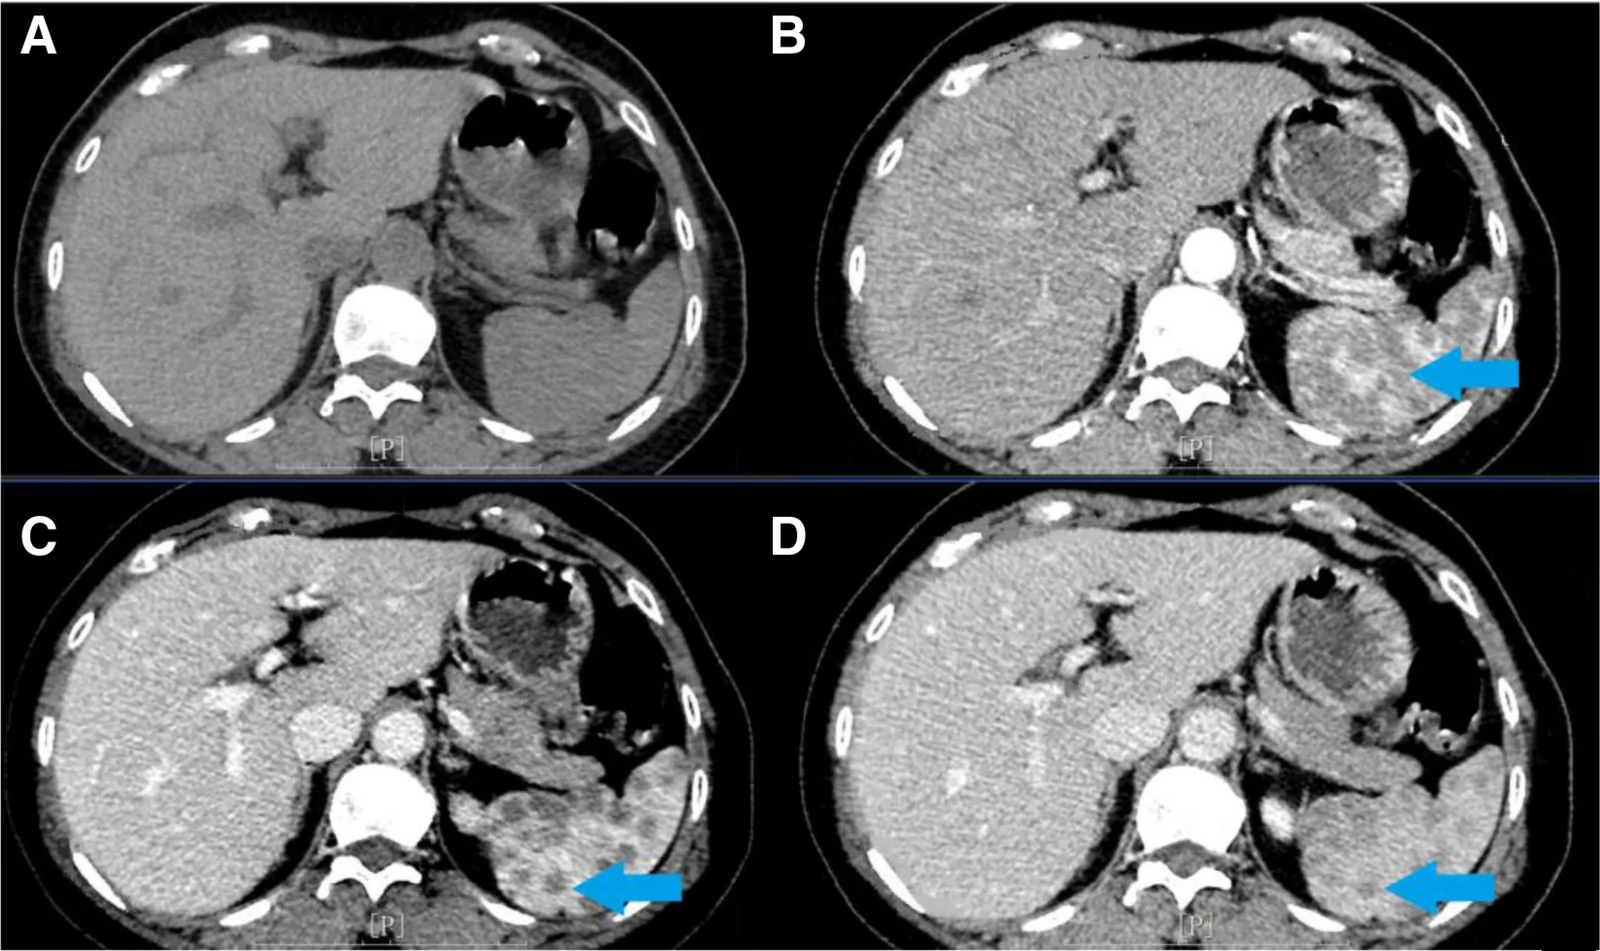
Abdominal CT scan showing a non-enlarged spleen with an irregular contour and multiple tiny hypodense nodules. (A) Unenhanced phase showing isodense nodules; (B) arterial phase showing hypodense nodules; (C) portal venous phase showing relative hypodensity; (D) equilibrium phase showing hypodense nodules. CT = computed tomography.
